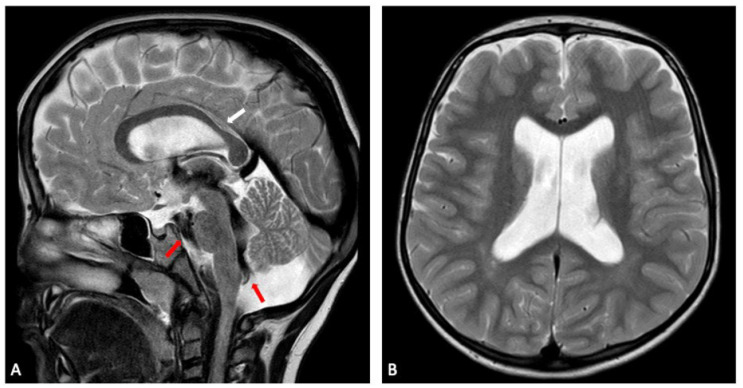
Sibling 1. Brain MRI; sagittal (A) and axial (B) T2-weighted turbo spin echo (TSE) images showing slight enlargement of the ventricular system. In (A), a prominent flow void (red arrows) is seen within the third ventricle, the cerebral aqueduct, the fourth ventricle and the prepontine cistern, expressing turbulent CSF flow, in keeping with no evident obstruction of CSF flow through ventricles and cisterns. Focal thinning of the posterior corpus callosum at the body-splenium junction can also be noted (white arrow in (A)).
